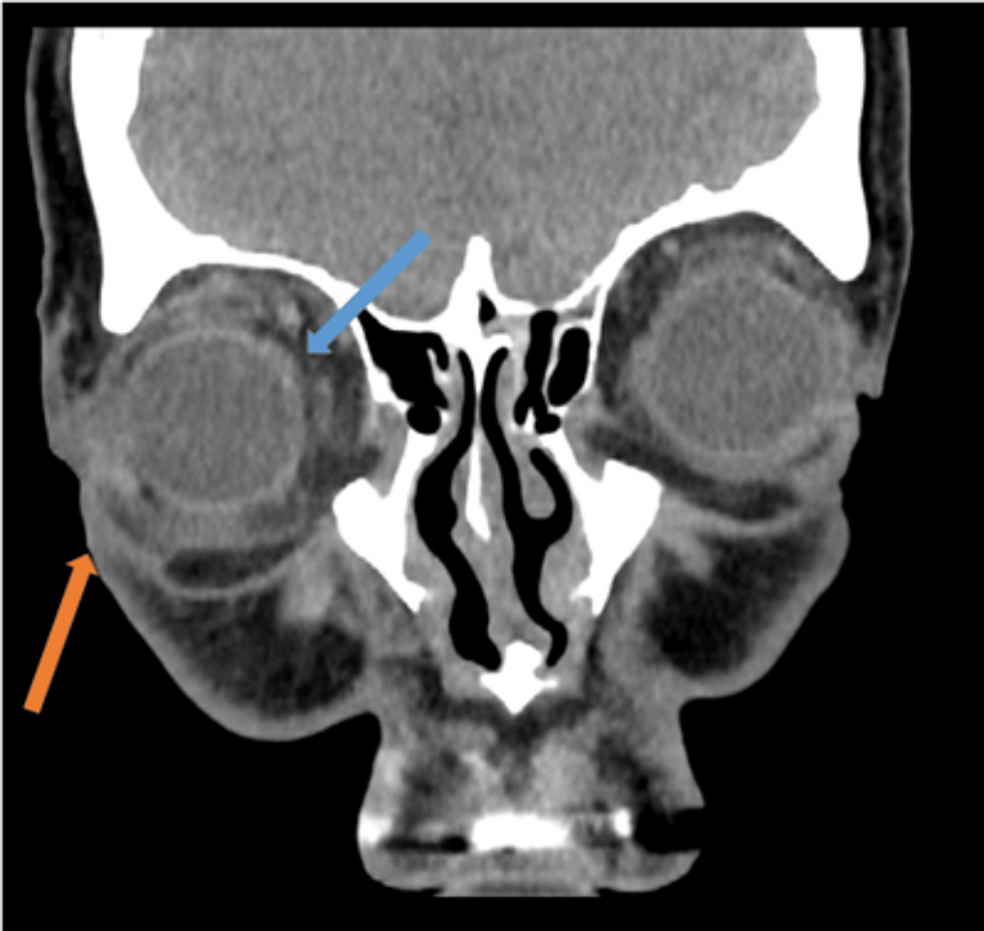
Contrast-enhanced CT orbits – coronal image Demonstrates orbital fat stranding (blue arrow) with significant periorbital oedema (orange arrow).
